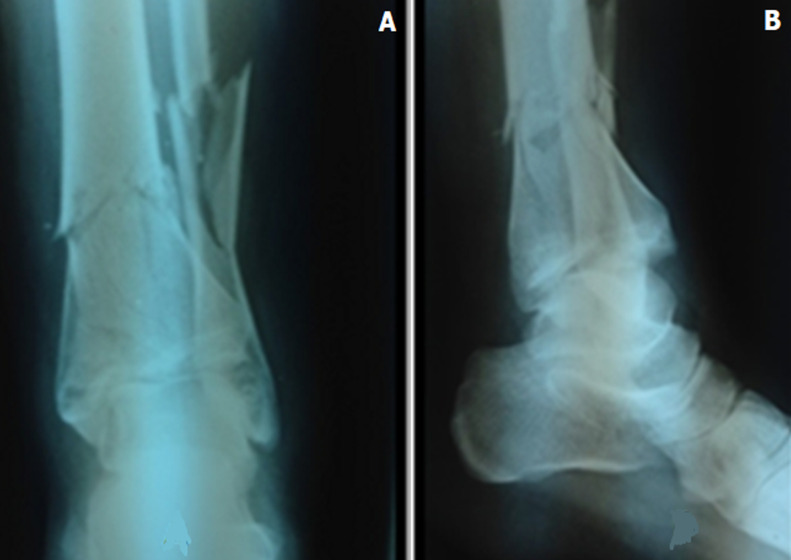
radiographie de la cheville face (A) et profil (B) montrant une fracture du pilon tibial droit Type C3 associé à une fracture de la malléole externe droite
